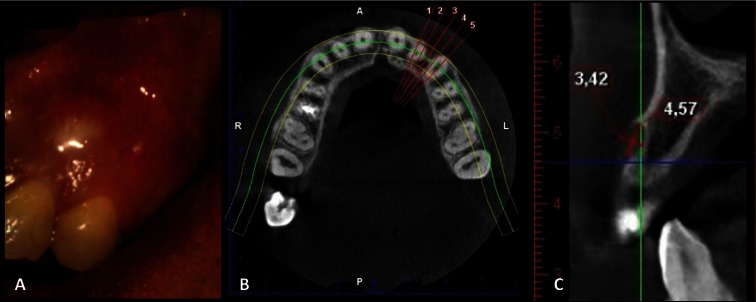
A) Intraoral view of the lesion B) CBCT: axial section and C) CBCT: sagittal section: lesion location, intraosseous and extraosseous involvement.
